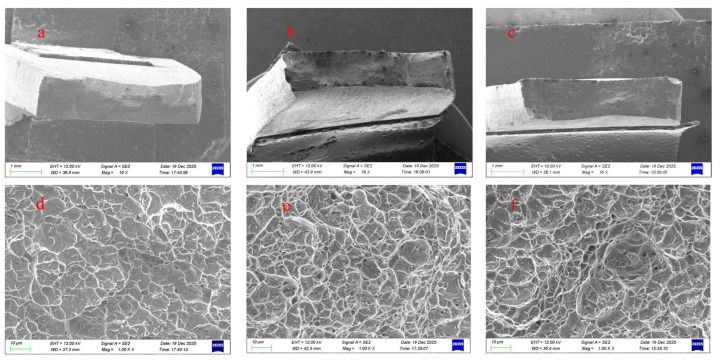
SEM images of tensile fracture surfaces under different ultrasonic frequency pulse currents: (a) 0 A, low magnification; (b) 6 A, low magnification; (c) 30 A, low magnification; (d) 0 A, high magnification; (e) 6 A, high magnification; (f) 30 A, high magnification.
